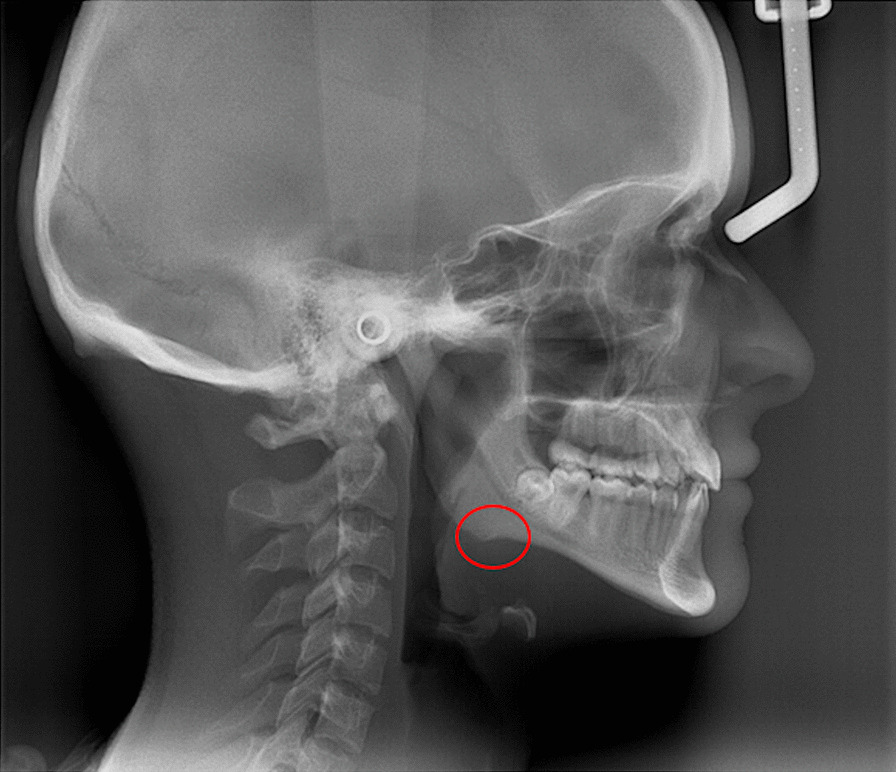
Lateral skull x-ray. The antegonial recess which is related to the pulsatile activity of the facial artery, is observed [23]
